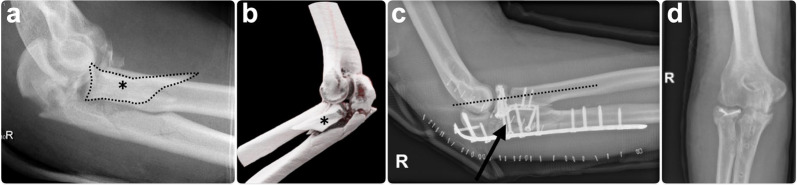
Example of radiographic follow-up of a trans-ulnar basal coronoid (TUBC) fracture–dislocation. a A 36-year-old male patient sustained a trans-ulnar basal coronoid fracture–dislocation of the elbow with a large basal coronoid fragment (dashed line) following a high-energy motor vehicle accident. b Three-dimensional CT reconstruction demonstrates displacement of the basal coronoid fragment (^*^) with associated ulnohumeral subluxation. c Postoperative radiographs after dorsal plate fixation of the ulna and additional deep lateral approach for radial head plate fixation show a centered radiocapitellar alignment without signs of persistent instability. In this case, the basal coronoid fragment was fixed through the plate with additional lag screws (arrow). d Radiographs obtained 18 months postoperatively, after implant removal, demonstrated post-traumatic osteoarthritis of the ulnohumeral joint
